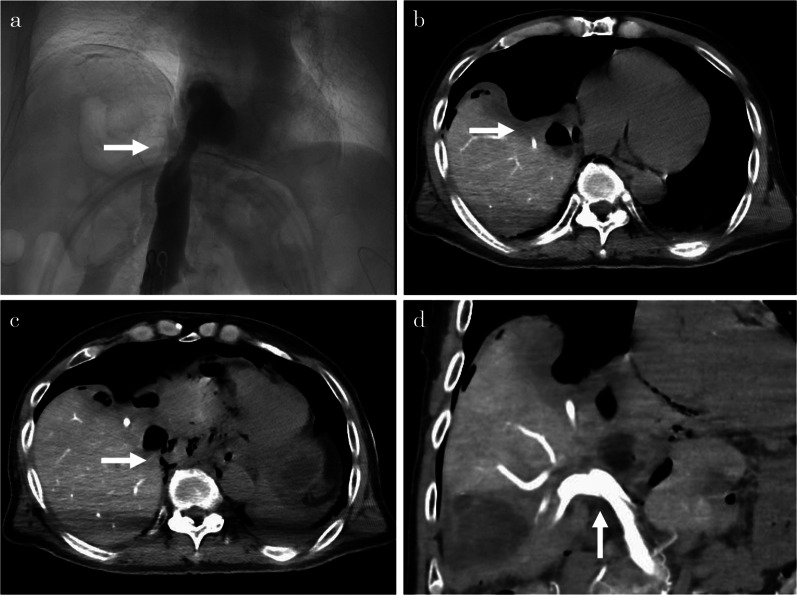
Intraoperative imaging studies. Angiography confirms that the stenosis of the inferior vena cava (IVC) was resolved after cyst fenestration (a). Computed tomography confirms that the cysts decompressed the right heart and that cyst fenestration released the IVC stenosis (b, c). Computed tomography arterial portography confirms that the stenosis of the portal vein had improved (d)
